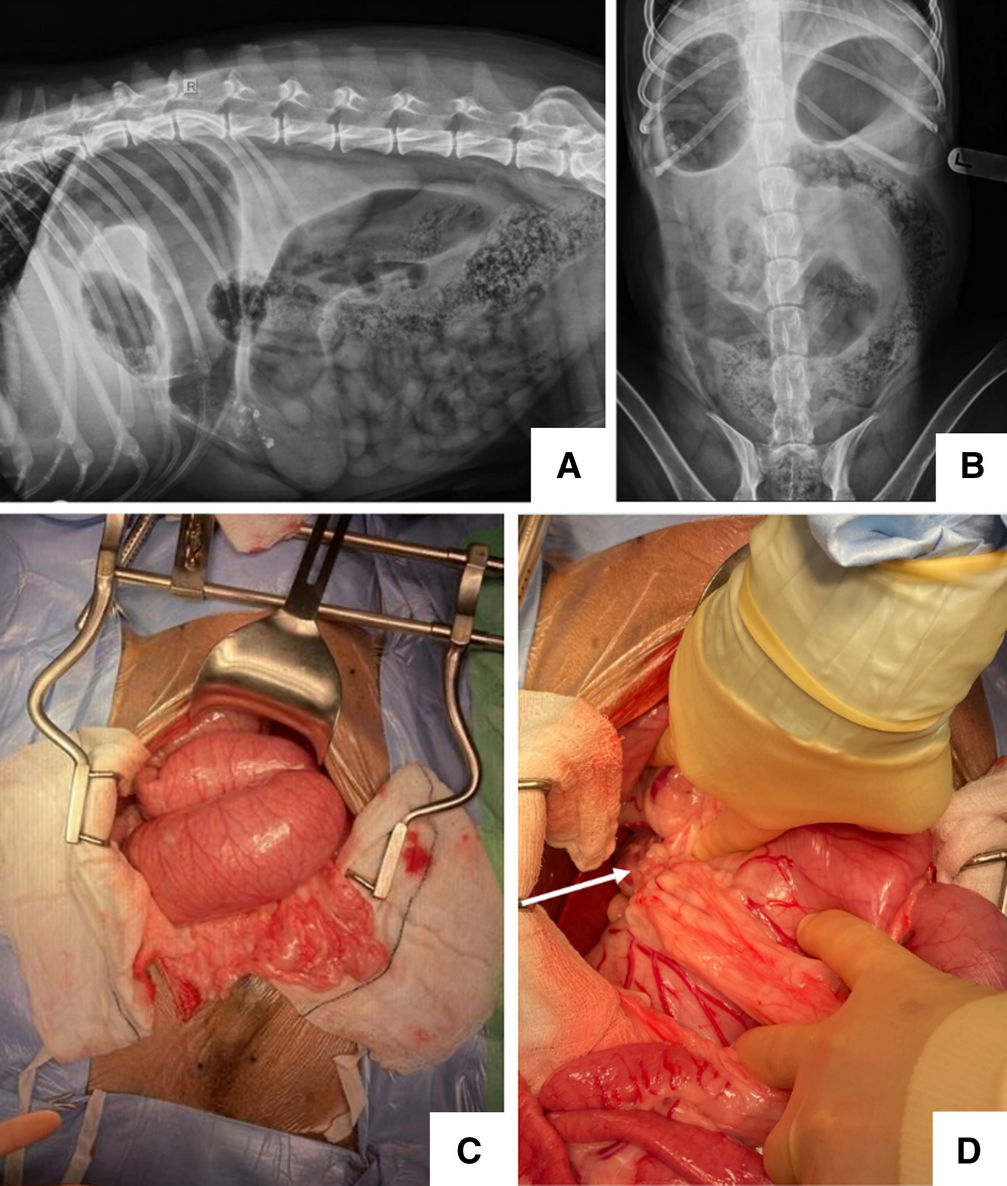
Right lateral (A) and ventrodorsal (B) abdominal radiographs. Intraoperative photos: marked duodenal distension (C) and omental adhesion (white arrow) (D).
